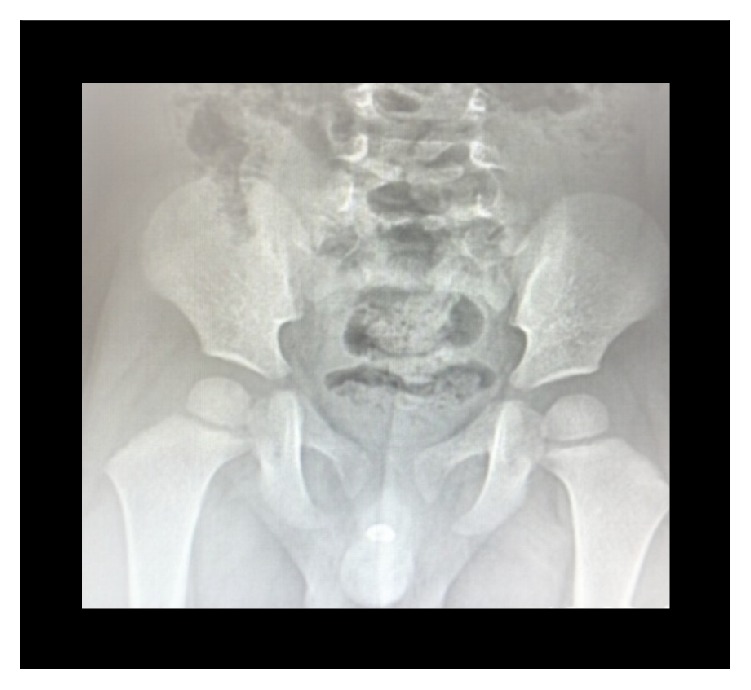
Plain abdominal radiograph showing the urethral calculus (case 1).
